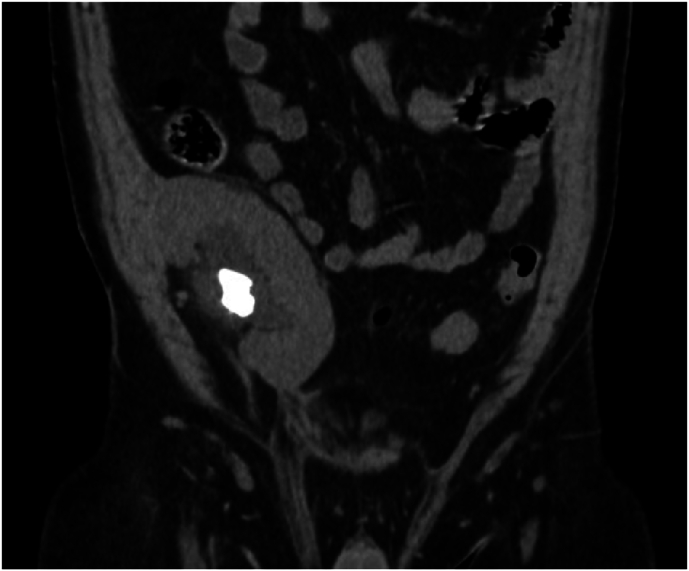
CT revealing a 2.6 cm staghorn calculi found in the transplanted kidney.
